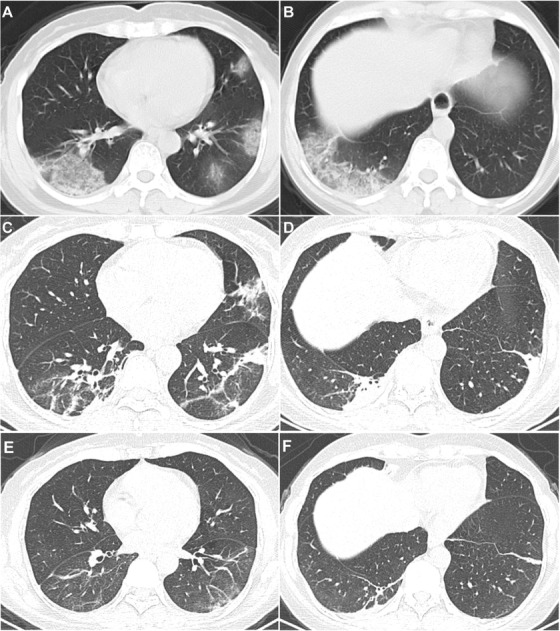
Chest computed tomography images. A and B, Subpleural exudation opacities in the lower right, left upper lung and left lower lung, on 2 February 2020. C and D, Fibrous lesions in the lower right, left upper lung, and left lower lung, on 11 February 2020. E and F, Fibrous lesions in the lower right, left upper lung and left lower lung, on 17 February 2020
